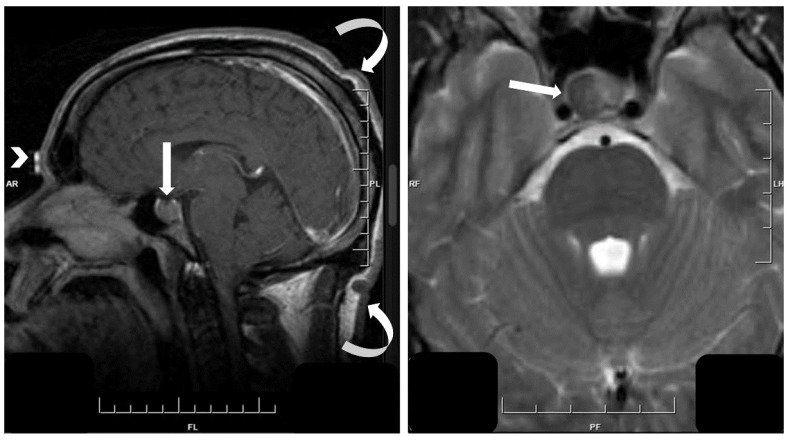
Magnetic resonance imaging of densely granulated somatotroph tumor. The sella turcica contains an intrasellar tumor (left, straight arrow); on T2-weighted imaging it is hypointense (right, straight arrow). Note the evidence of acromegaly in the thickened wavy skin (left, curved arrows) and frontal bossing (left, chevron).
